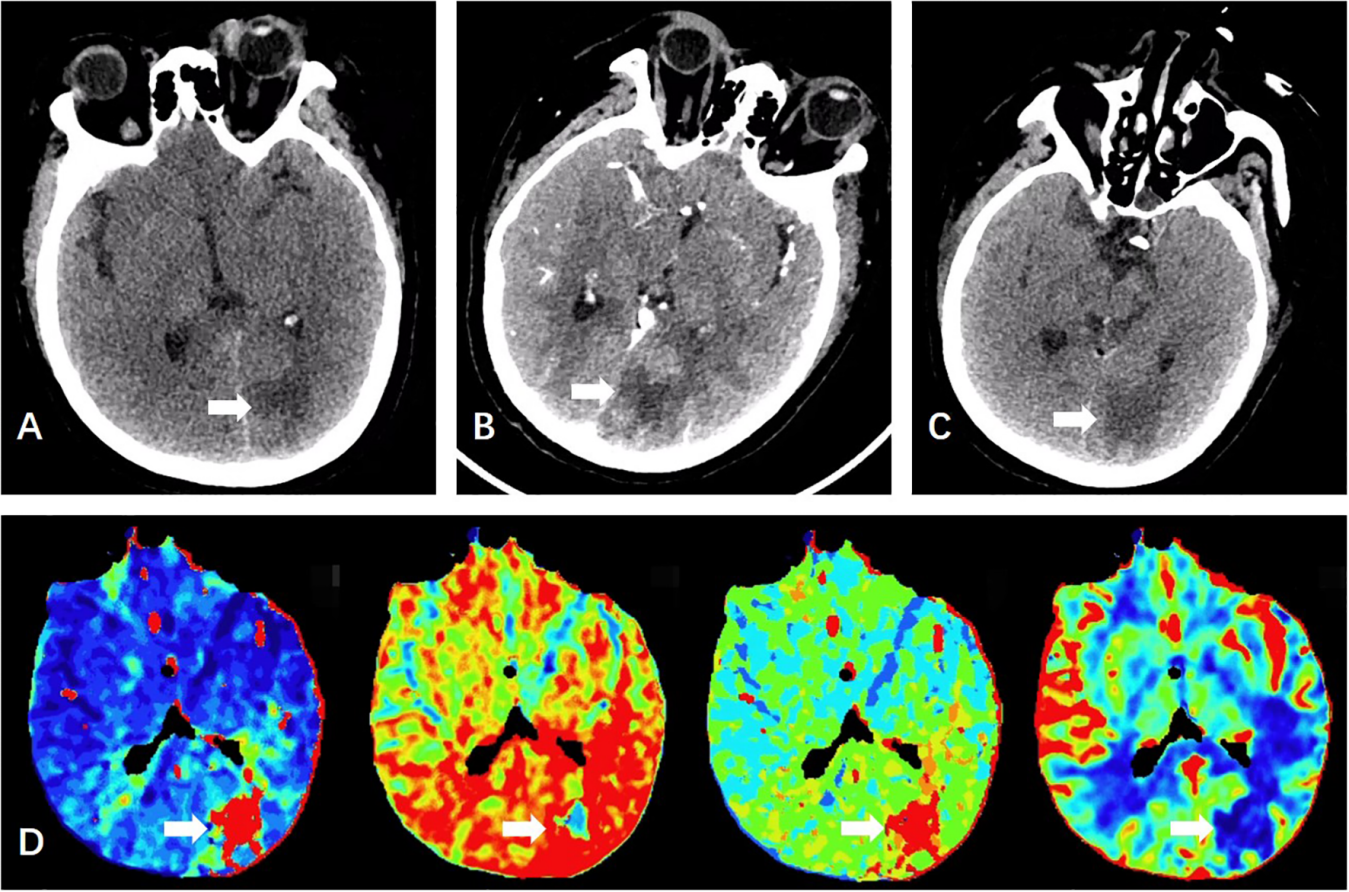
Initial non-contrast head CT (2025-08-19) reveals an irregular patchy hypodensity in the left parieto-occipital lobe (A). Perfusion imaging shows reduced CBF, reduced CBV, reduced MTT, and prolonged TTP in the left parieto-occipital hypodense area: the volume with rCBF < 30% is approximately 0 ml, and the volume with Tmax > 6s is approximately 33 ml (D). Contrast-enhanced head CT (2025-08-22) demonstrates the irregular patchy hypodensity in the left parieto-occipital lobe with ill-defined margins, showing no significant enhancement post-contrast (B). A few small soft tissue nodules are noted in the left frontal and right submandibular subcutaneous regions, as well as bilaterally around the orbits, with the largest measuring approximately 9 mm in diameter. Follow-up head CT one week after treatment (2025-08-26) shows multiple irregular patchy hypodensities in the bilateral parieto-temporo-occipital lobes and the left frontal lobe. Compared to the previous scan, the involved areas have increased in extent, with some lesions being newly developed (C).The lesion is shown as the white arrow in the picture.
